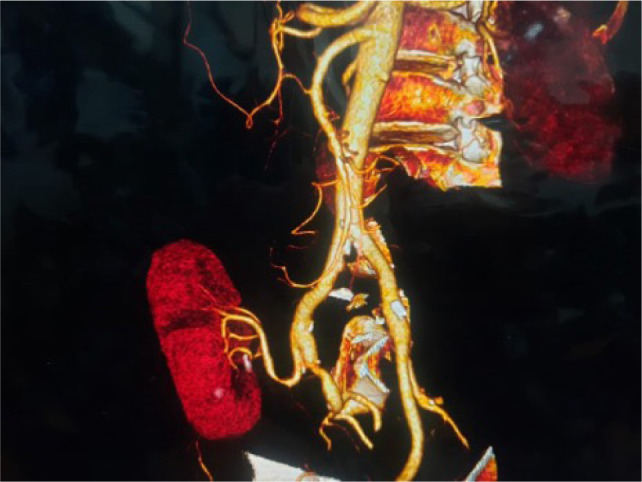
Three-dimensional reconstruction of computed tomography angiogram post autotransplantation displaying successfully transplanted kidney with clear visualization of the vascular anastomosis.
